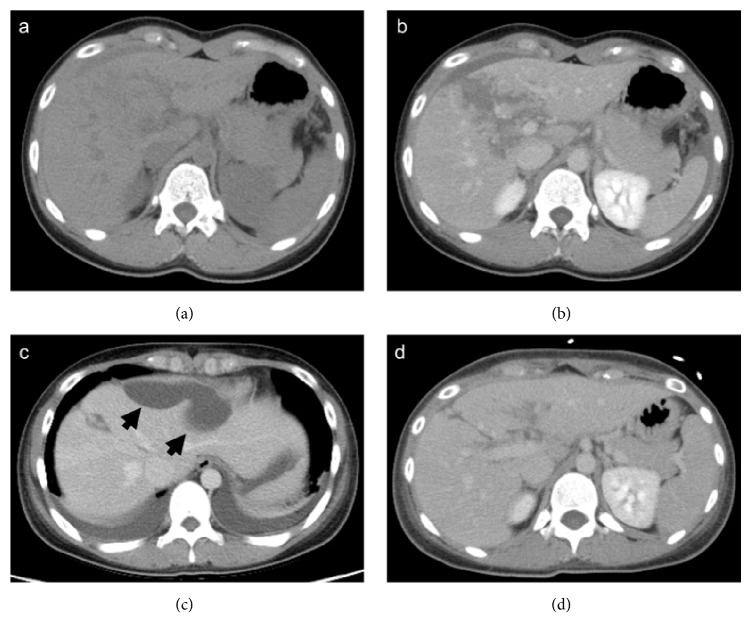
(a) Computed tomography (CT) performed on arrival at the hospital showing hepatic injury. (b) Enhanced CT performed on arrival at the hospital showing hepatic injury. (c) Enhanced CT performed on day 17 of hospitalization showing biloma (black arrows). (d) Enhanced CT performed on day 34 of hospitalization showing that the biloma had almost disappeared.
